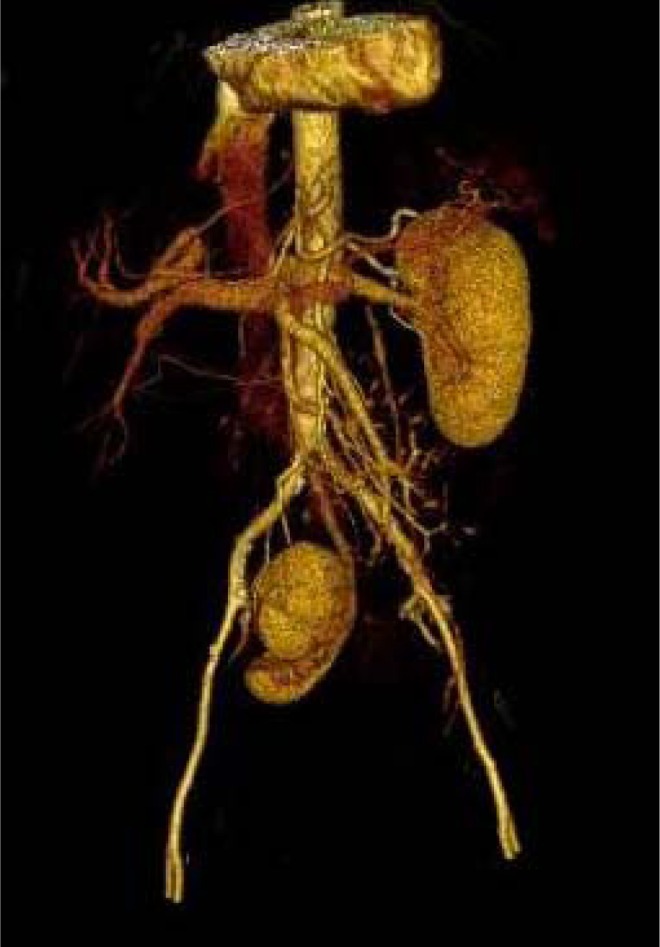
CT angiography of the abdomen and pelvis with 3D reconstruction displaying a single right renal artery originating from proximal right common iliac artery.
